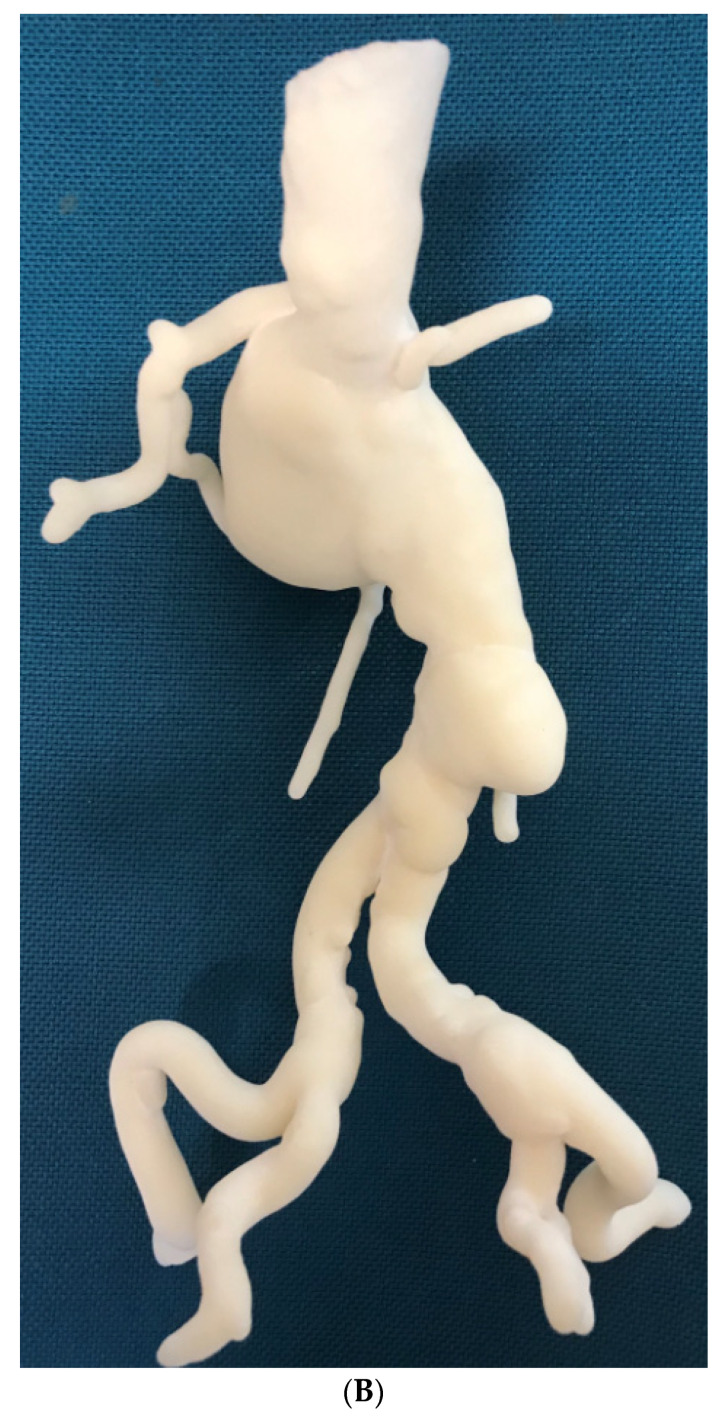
3D printed model of abdominal aortic aneurysm from CT angiographic data. (A) Anterior review of the 3D printed AAA model showing an infrarenal AAA extending to common iliac arteries. (B) Posterior view of the aneurysm and renal arteries. The mode was printed with ‘Strong and Flexible Plastic’ material by Shapeways.
